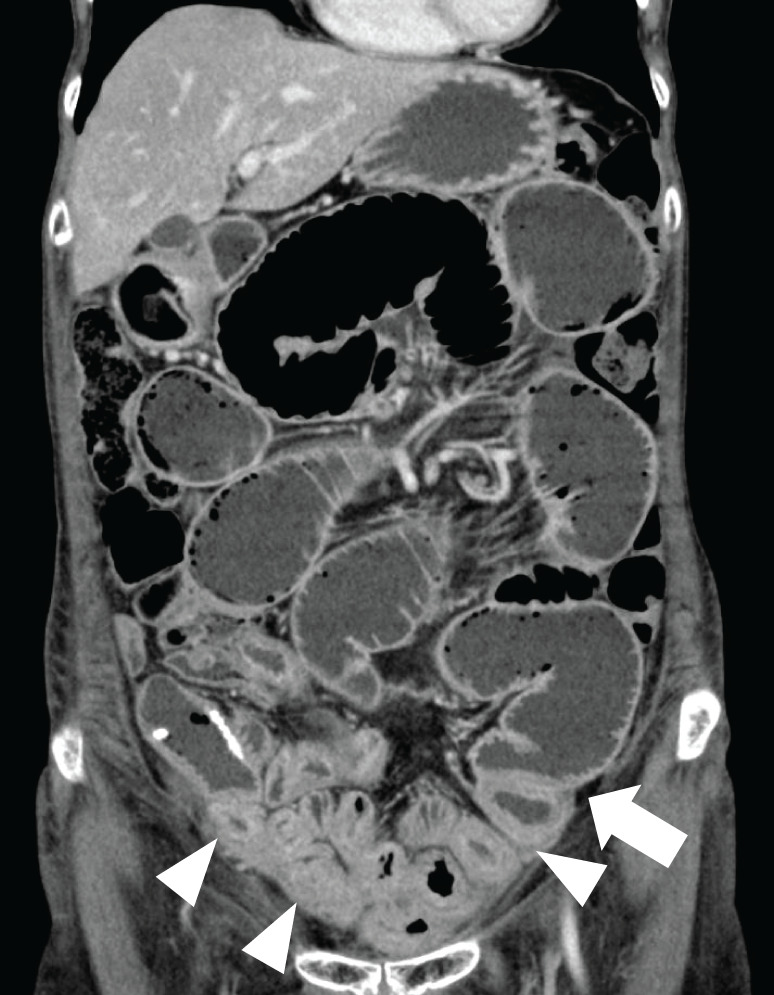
Coronal view of abdominal CE-CT at the initial visit represents wall thickening (arrowhead) and caliber change (arrow) of the small intestine. CE-CT, contrast-enhanced CT
